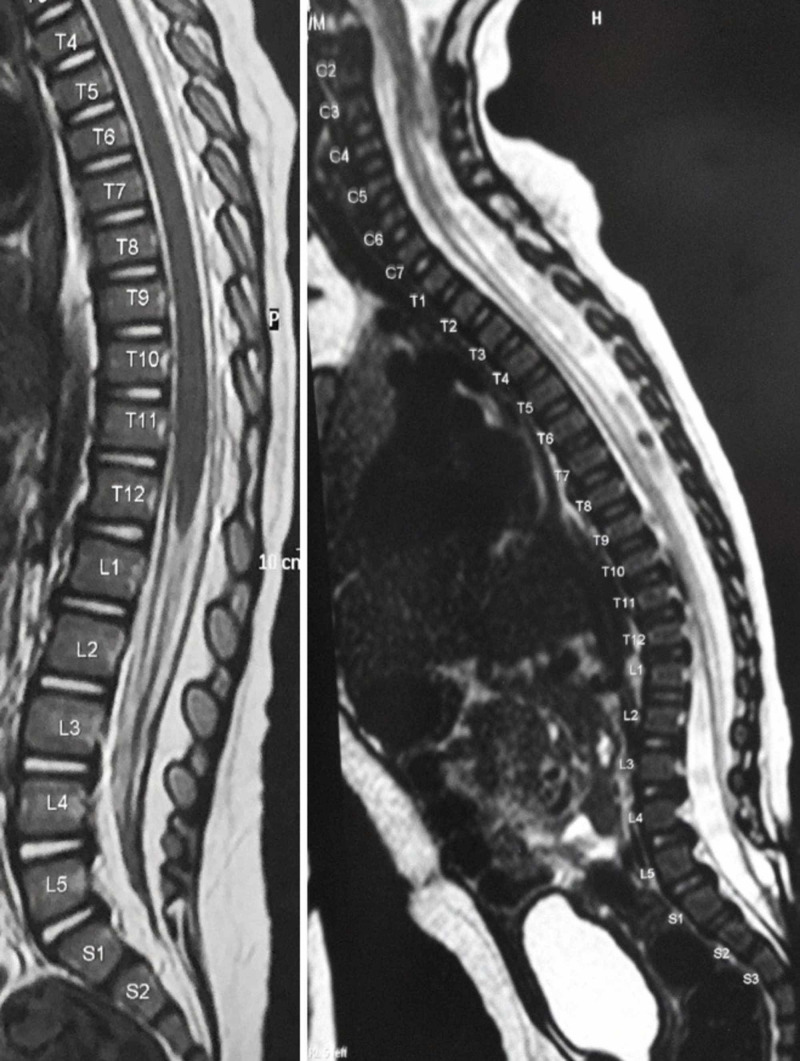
Normopositioning of conus medullaris and standard radiological findings of secondary tethered cord syndrome Normopositioning of the conus medullaris at the level of T12 on a sagittal T2-weighted spinal MR image (left) and low-lying position of the conus medullaris at S3 in a four-month-old child who underwent post-natal MMC repair and had no clinical symptoms of tethered cord syndrome (right) MMC: myelomeningocele
